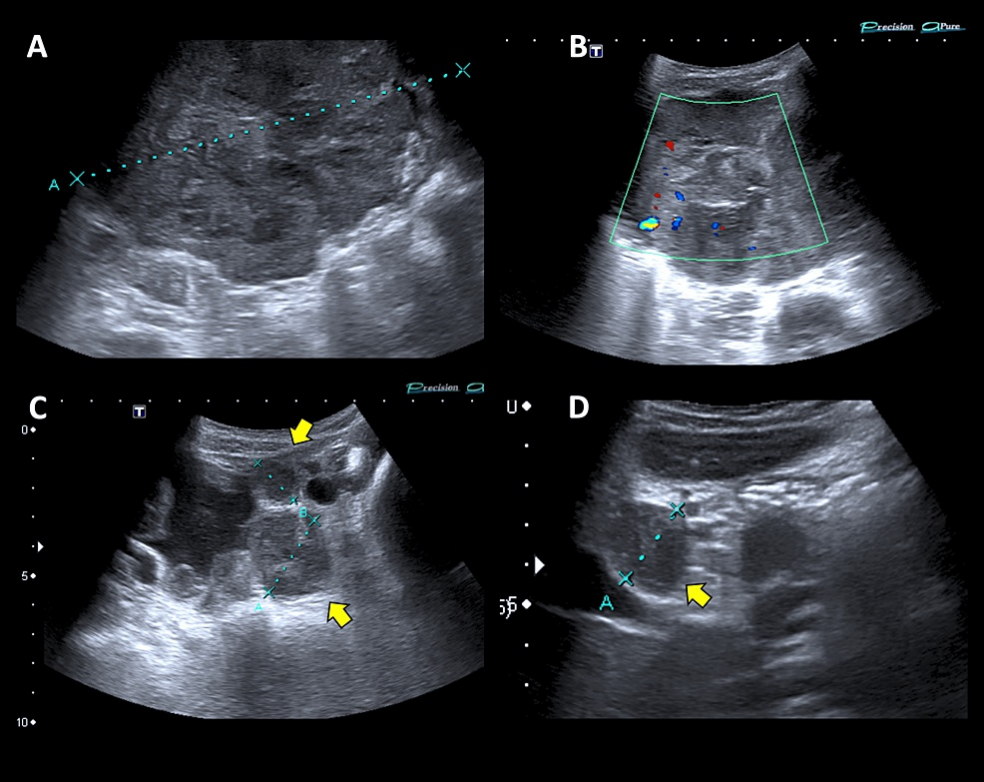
Ultrasound A, dominant mass is present on the right side of the abdomen with central vascularization and a diameter of up to 13 cm; C-D, multiple smaller peritoneal implants are found in the lower quadrants (arrows)
